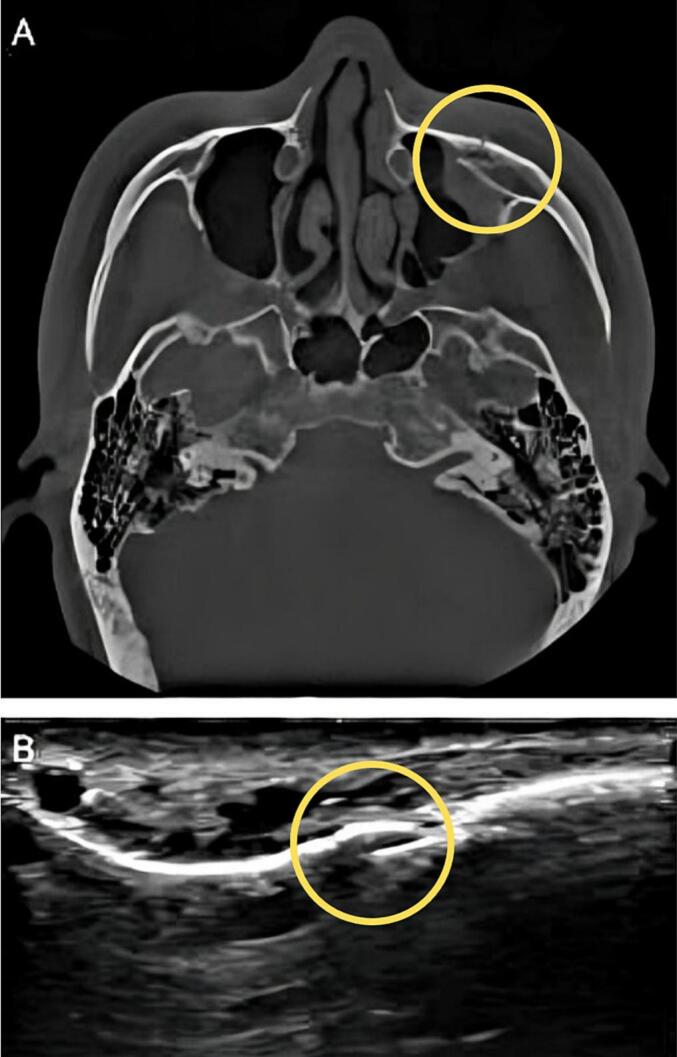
A: CBCT measurement of orbital rim displacement. a: medial fragment of orbital rim. b: lateral fragment of orbital rim. B: Ultrasonographic measurement of orbital rim displacement for the same patient. a: medial fragment of orbital rim. b: lateral fragment of orbital rim. star: infraorbital foramen. Yellow circle: fracture site and measurement of fracture displacement. (For interpretation of the references to colour in this figure legend, the reader is referred to the web version of this article.)
